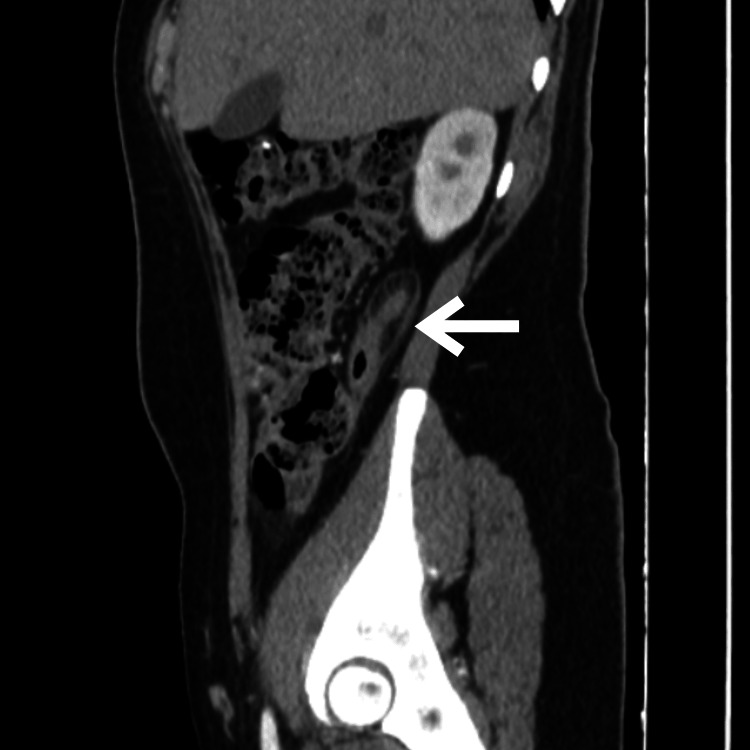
Computed tomography scan showing a dilated appendix, sagittal view (white arrow).
